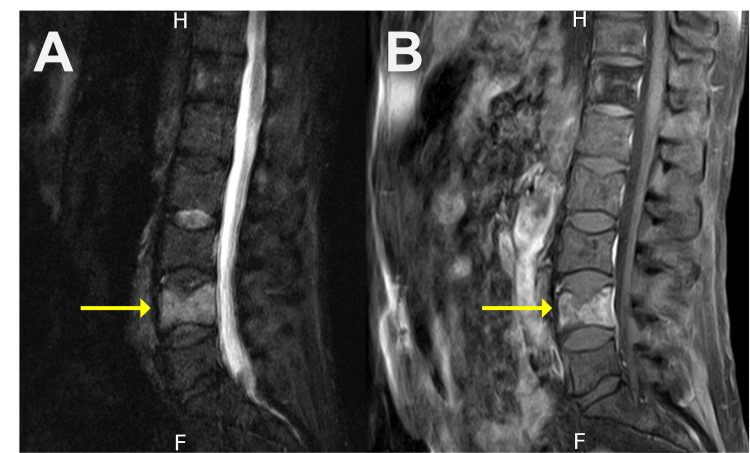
(A) Sagittal short tau inversion recovery and (B) sagittal T1-weighted fast spin echo with fat saturation and intravenous contrast magnetic resonance imaging showing an acute L4 vertebral body fracture with 40% height loss (yellow arrows)
